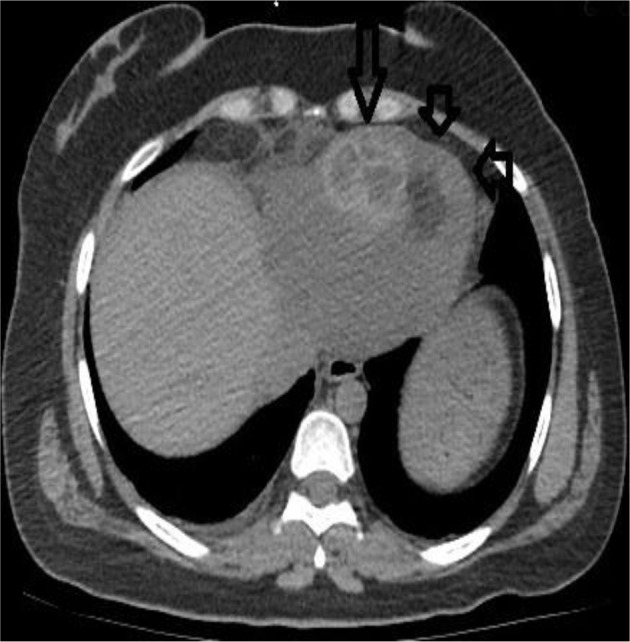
Axial CT image at the second admission demonstrates pericardial effusion (short arrows) associated with hydatid lesion (Long arrow)
